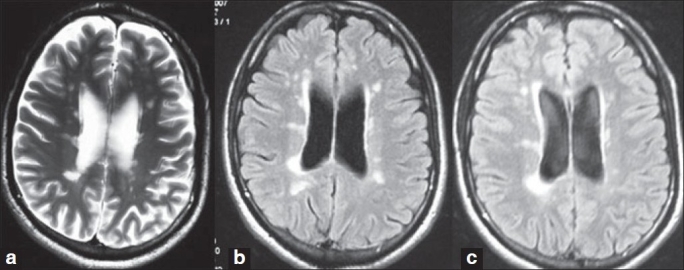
(a) Baseline (2006) MRI brain of one our patients with RRMS before starting IFN β. (b) Follow up (2007) MRI brain of patient showing no fresh lesion (c) Follow up (2008) MRI brain of patient showing no fresh lesion
